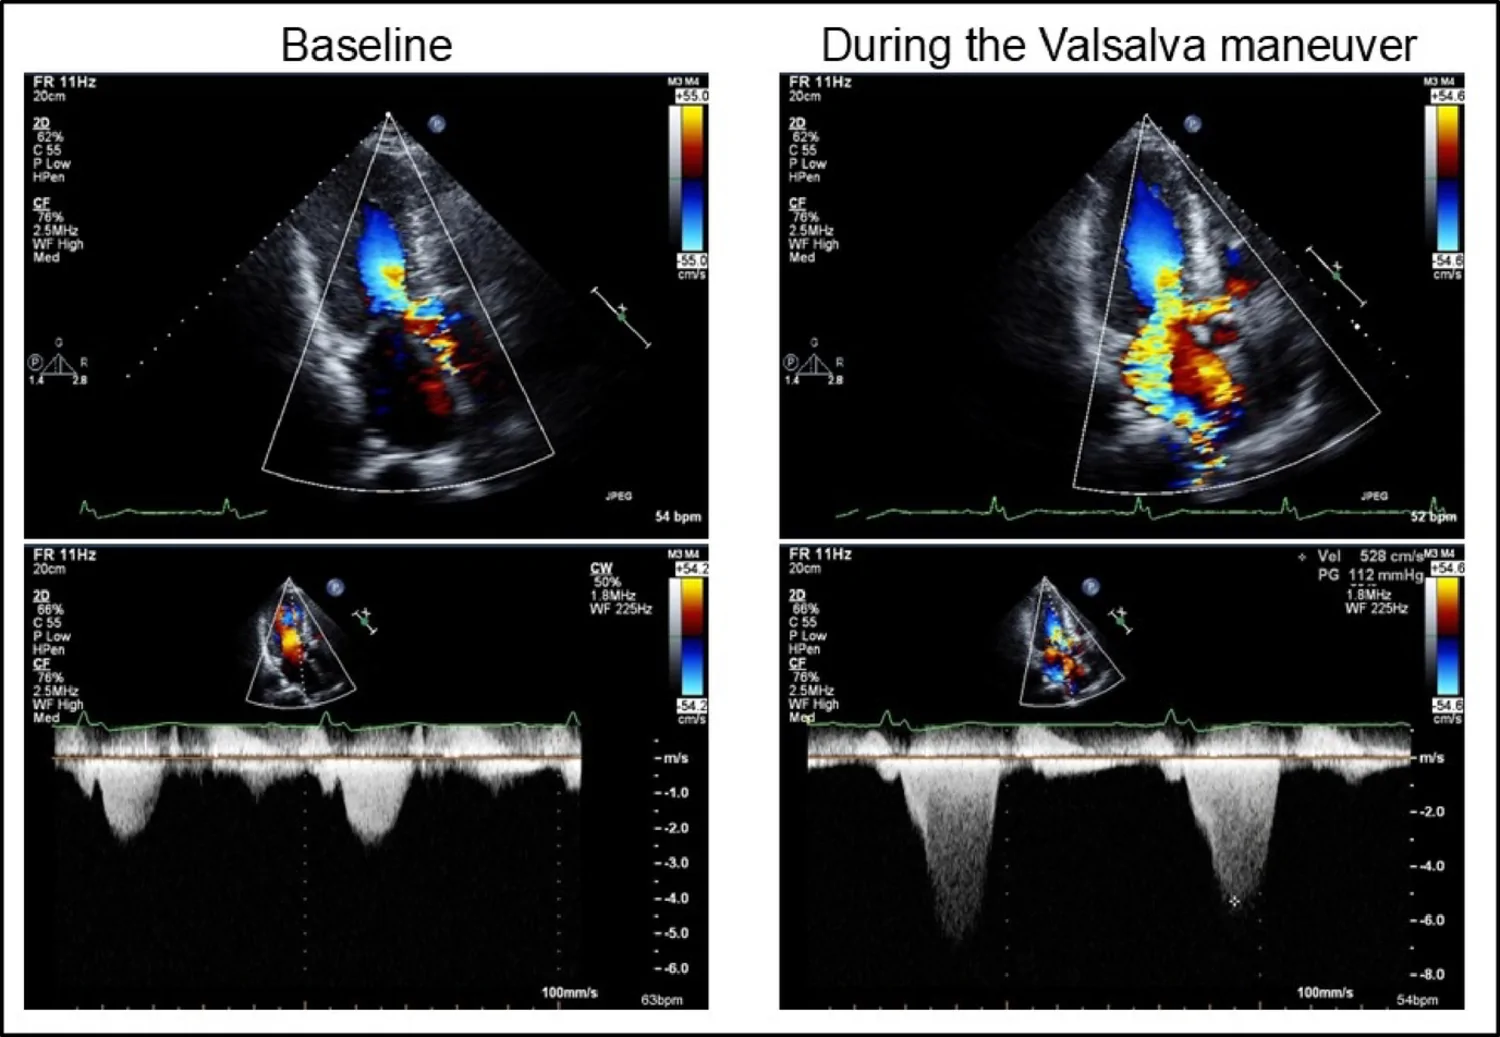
Valsalva maneuver for latent left ventricular outflow tract obstruction. Apical long-axis color Doppler image (top) and a continuous-wave Doppler image of the left ventricular outflow tract (bottom). The Valsalva maneuver causes mitral regurgitation associated with the systolic anterior motion of the mitral valve, increasing the mosaic flow in the left ventricular outflow tract. Continuous-wave Doppler imaging, which avoids mitral regurgitation, showed a pressure gradient of 112 mmHg, indicating outflow tract obstruction
